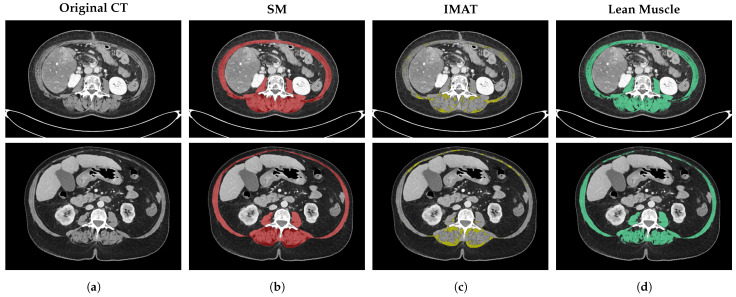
Qualitative assessment of skeletal muscle radiodensity and myosteatosis characterization. (a) The original CT image at L3 level; (b) the total skeletal muscle boundary predicted by FocusedON-BC in red; (c) the intermuscular adipose tissue (IMAT) mask extracted via radiodensity thresholding (<−30 HU) in yellow; and (d) the resulting lean-muscle mask (>=−30 HU) in green.
